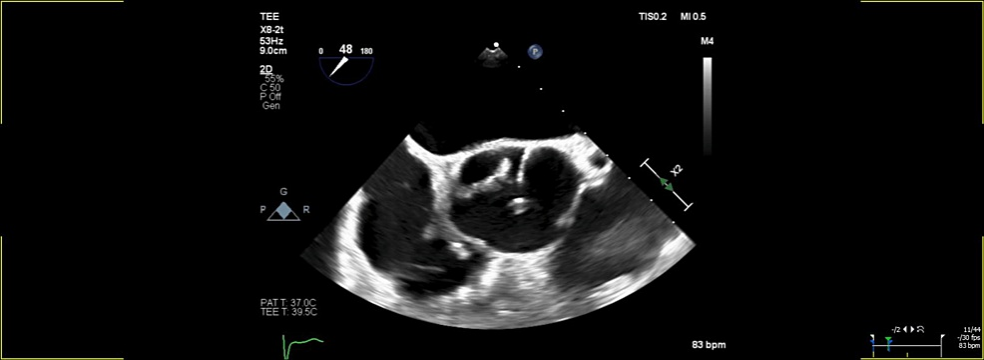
Echocardiogram showing severe AR, prompting consideration of valve replacement AR: Aortic regurgitation
